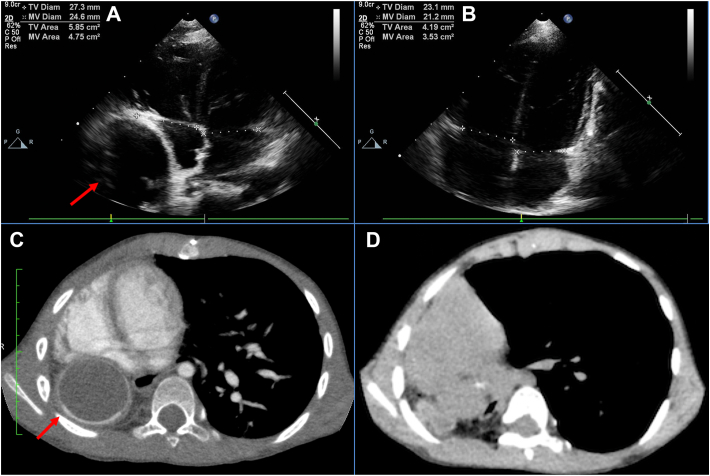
Transthoracic echocardiography (A, B) and computed tomography (C, D) before (left: A, C) and after (right: B, D) expander deflation. Arrows indicate the tissue expander.
